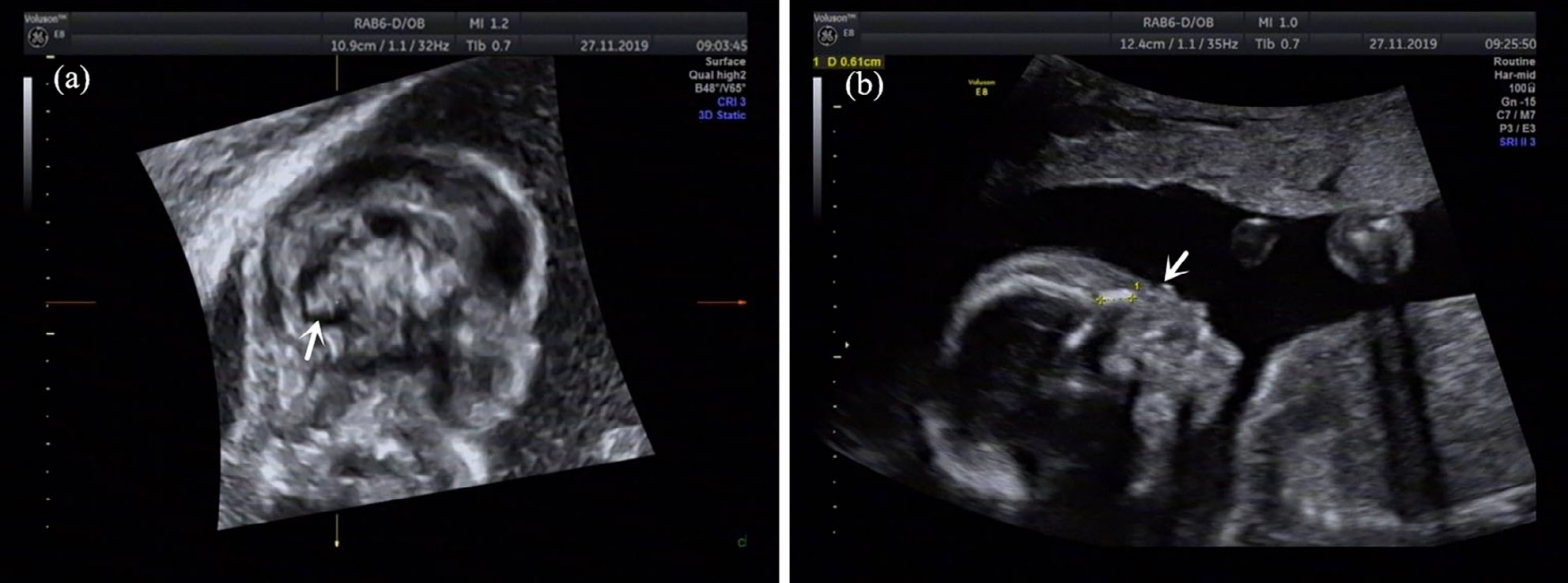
The fetal ultrasound imaging showed hypoplasia of the cerebellar vermis (a) and a flat nose (b).
